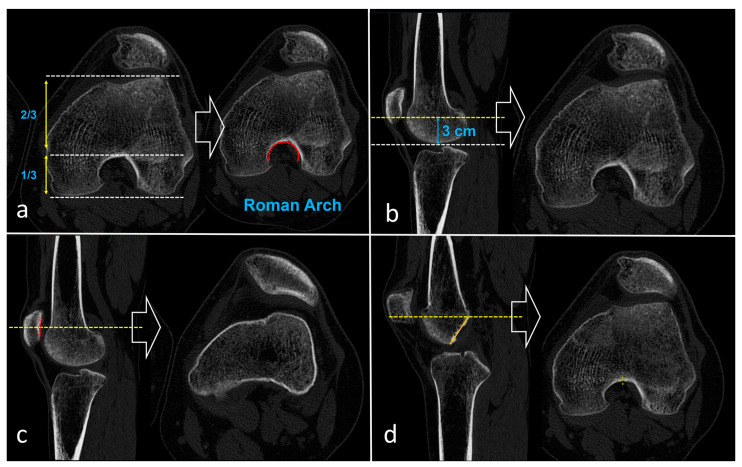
Determination of the four axial CT levels used for Dejour classification. (a) Roman arc level (Level 2): on the sagittal image, the distal femur is divided into a lower one-third and upper two-thirds, and the axial slice is chosen where the intercondylar notch resembles a Roman arch (red arc). (b) “3 cm above the joint line” level (Level 3): on the sagittal plane, a line is drawn 3 cm above the joint line (blue bracket), and the corresponding axial slice is obtained. (c) Midpatellar level (Level 1): the axial image is taken at the level passing through the midpoint of the patella on the sagittal reconstruction. (d) Top of the Blumensaat line level (Level 4): the axial slice is selected at the level tangent to the most superior point of the roof of the intercondylar notch (yellow line), corresponding approximately to the MPFL femoral footprint and the superior trochlea.
